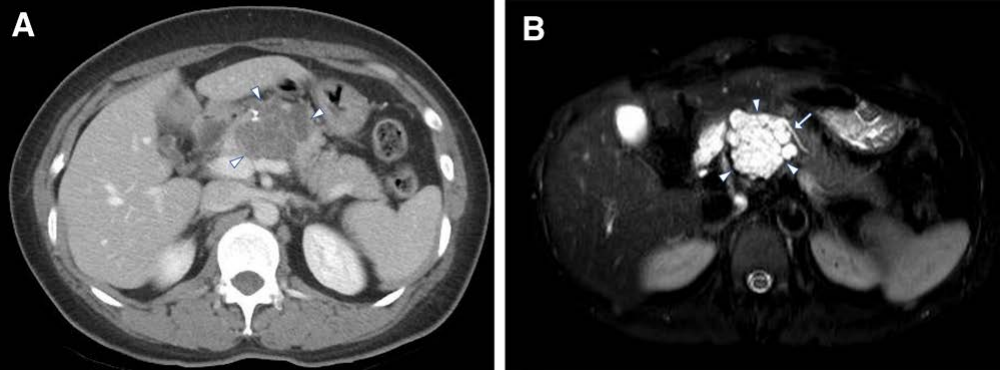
(A) Initial abdominopelvic computed tomography revealed a multilobulated low-density lesion (arrowheads) of approximately 5 cm with multiple enhancing septations and calcifications on pancreatic body. (B) Pancreas magnetic resonance imaging revealed a multiloculated cystic lesion (arrowheads) at the body of pancreas which appeared to be communicated with the main pancreatic duct (arrow).
